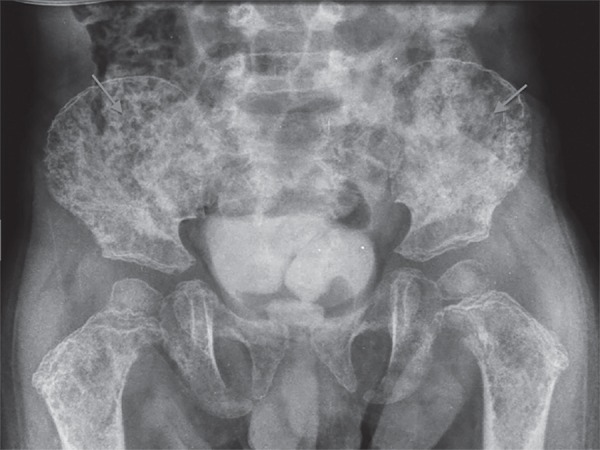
Pelvic X-ray showing osteolytic lesions (arrows).
